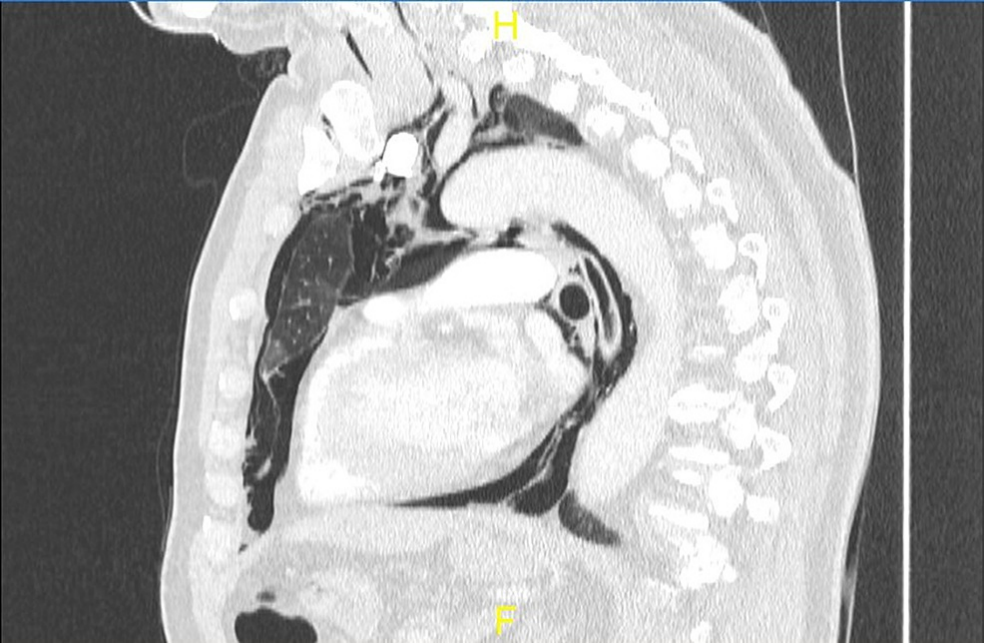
Sagittal CTPA slice demonstrating pneumomediastinum CTPA: computed tomography pulmonary angiogram
